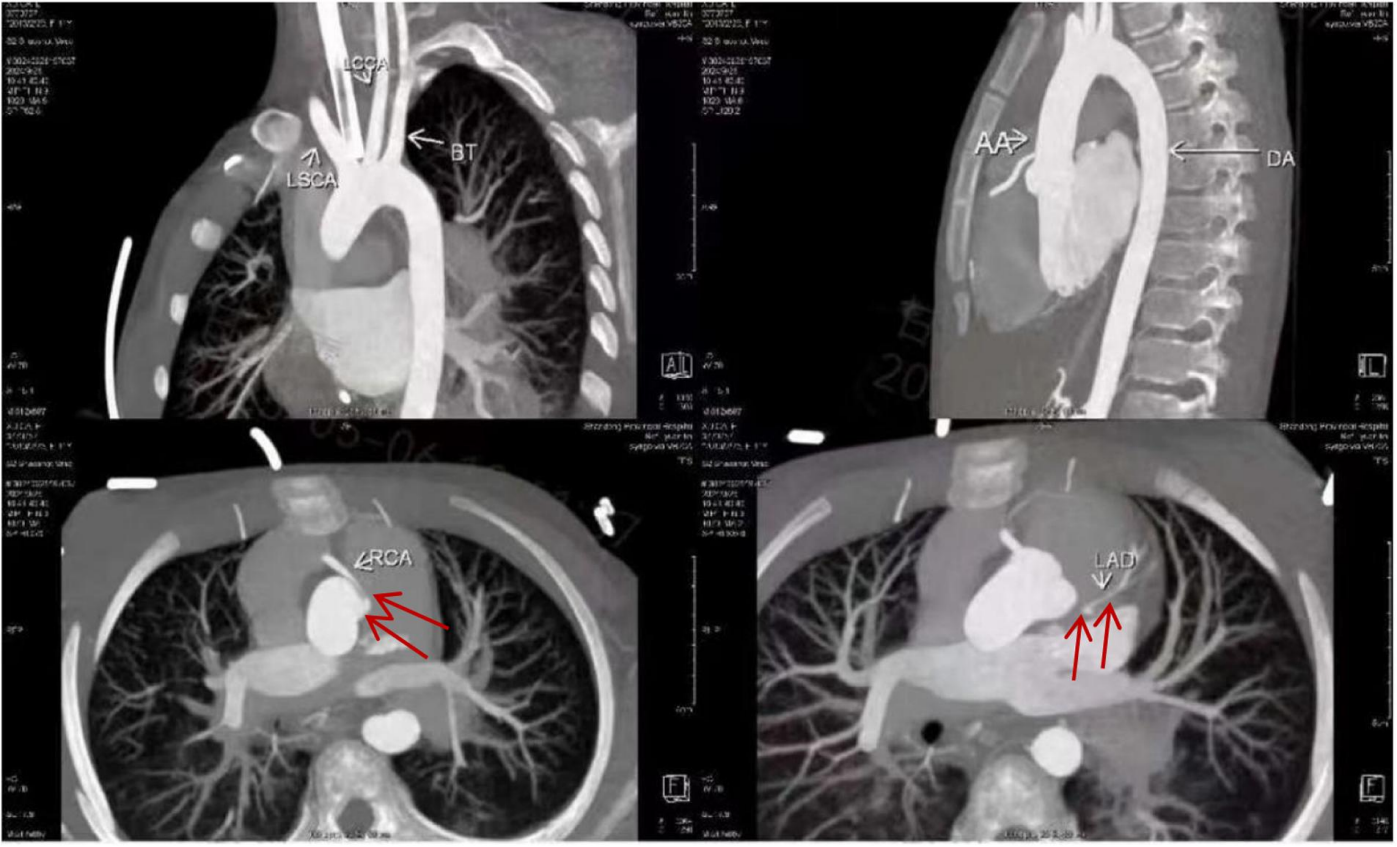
Coronary CTA shows the right coronary artery originates from the left coronary sinus and the left coronary artery and its branches exhibit slender luminal caliber (marked by a red arrow).
